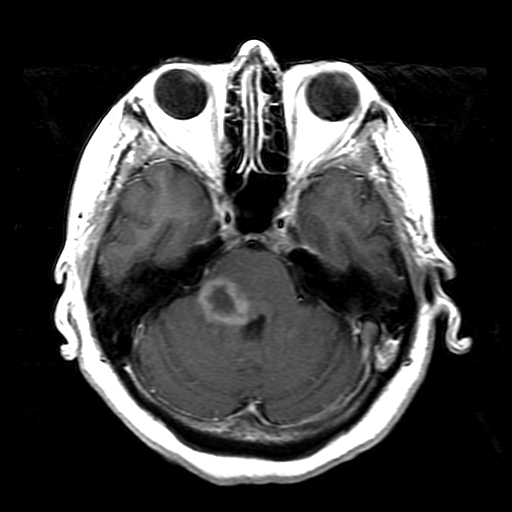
A 54 year old woman with auto-immune hepatitis on treatment with azathioprine for five years who presented with sub-acute neurologic symptoms of right facial drooping and loss of vision in the right eye. MRI revealed a ring-enhancing pontine lesion. Pathological examination proved this to be large B-cell primary central nervous system lymphoma (PCNSL). The imaging characteristics of the tumor were that of a ring-enhancing mass typical of PCNSL found in HIV patients.
